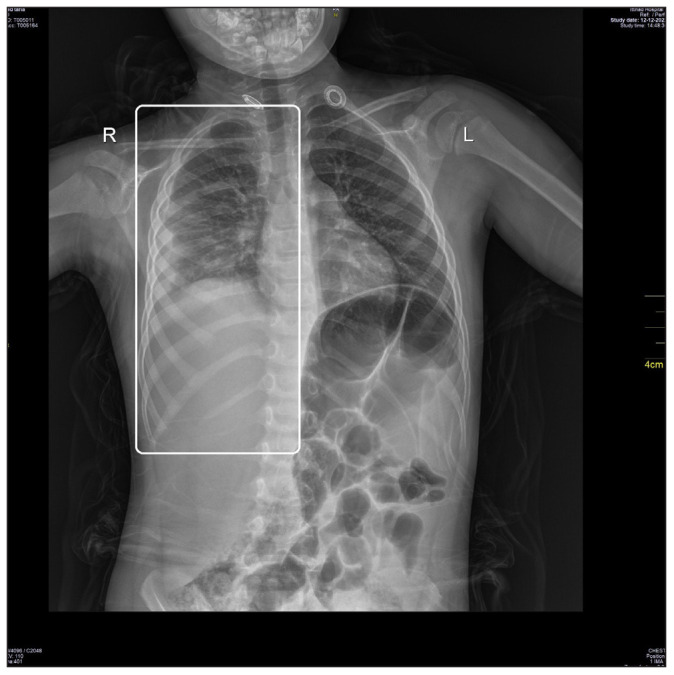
The patient’s initial X-ray image demonstrating a right-sided lower lobe pneumonia (white rectangle).
